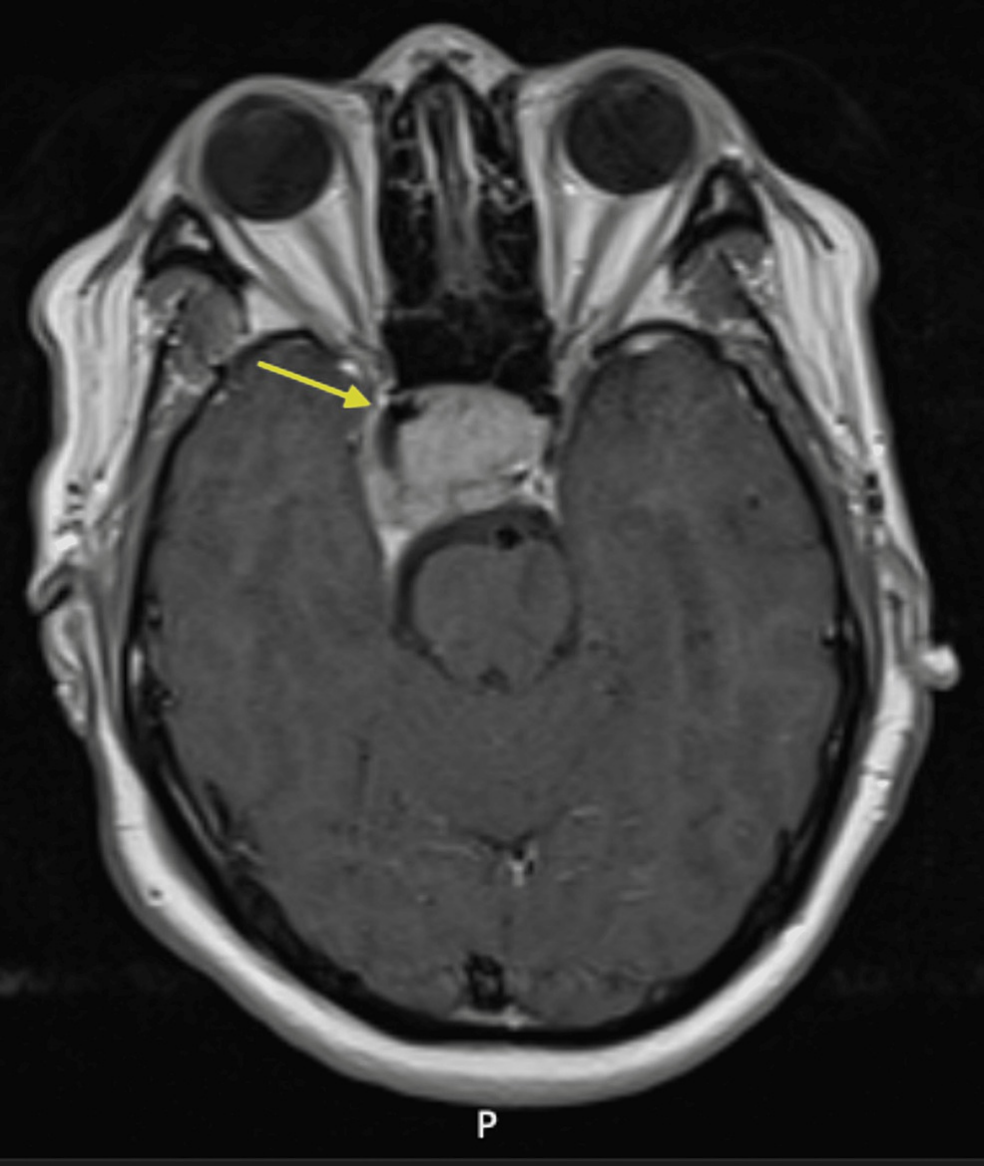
Axial MRI brain T1 SE post-contrast showing tumor around right internal carotid artery (yellow arrow).
